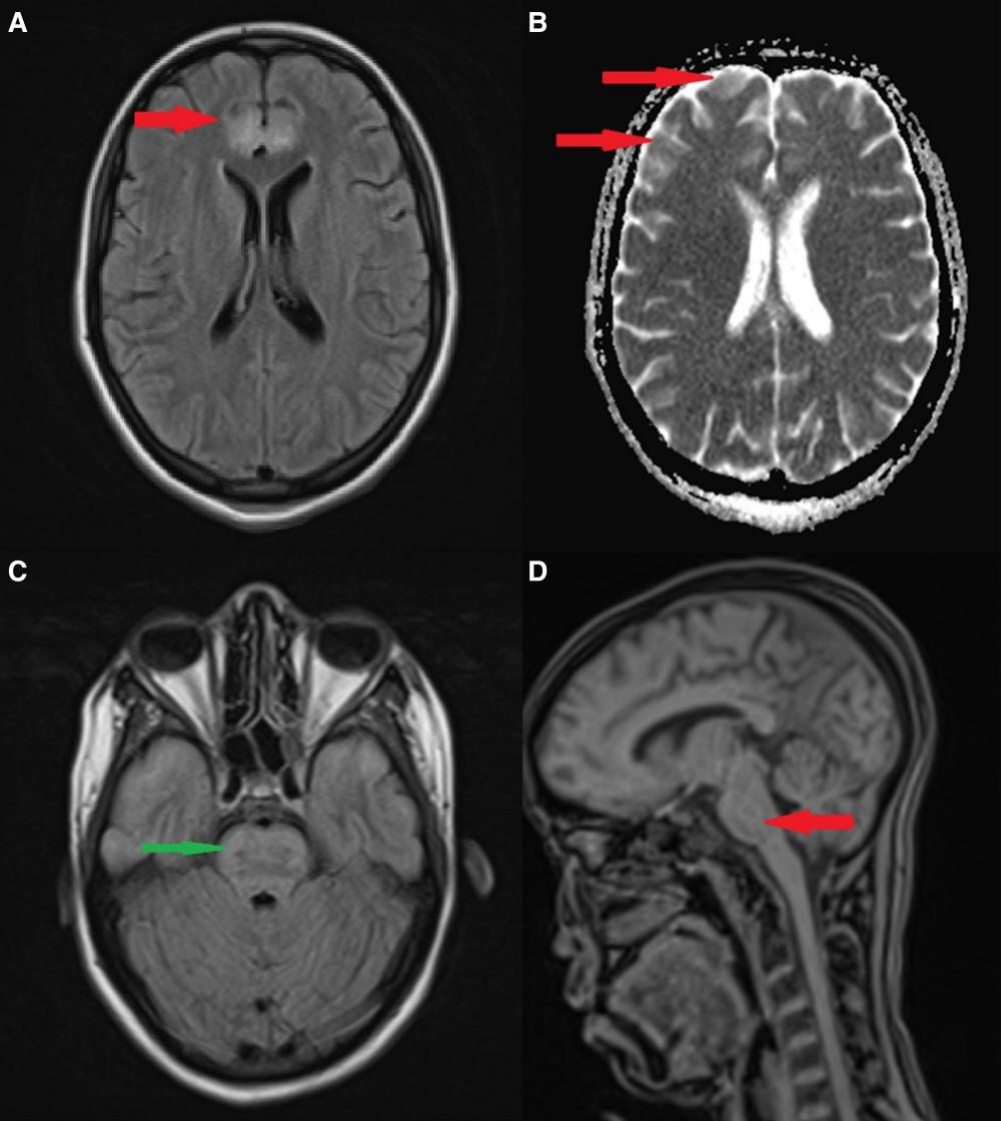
Magnetic resonance imaging (MRI) findings in a patient with diabetic ketoacidosis (DKA)-associated brain injury. A, Axial fluid-attenuated inversion recovery MRI showing cortical and subcortical hyperintensity in the left frontal lobe (arrow), suggestive of cerebral edema or ischemic injury. B, Axial diffusion-weighted imaging showing areas of diffusion restriction (arrows), consistent with cytotoxic edama and potential ischemic injury. C, Axial T1-weighted MRI demonstrating involvement of the brainstem (arrow), which may indicate hypoxic-ischemic injury or osmotic demyelination. D, The brainstem lesions are not enhanced after gadolinium injection. MRI findings were highly suggestive of ischemic pathway for brain damage. The reversible character as well as the rapid clinical recovery confirm the metabolic origin.
